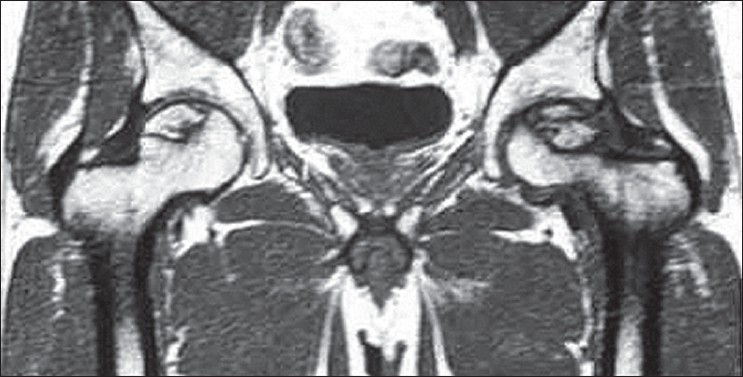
Preoperative MRI (T1 weighted images) of both hips showing bilateral avascular necrosis
